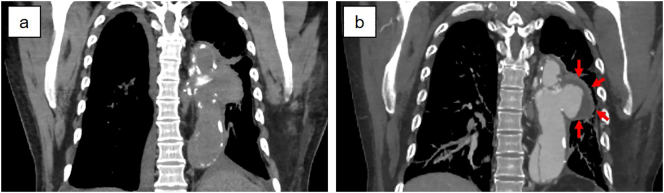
Computed tomography findings of the aortic aneurysm. a A cystic aortic aneurysm associated with a previous aortic arch replacement was present prior to the splenectomy. b Repeat computed tomography performed postoperatively reveals enlargement of the aneurysm (arrows).
